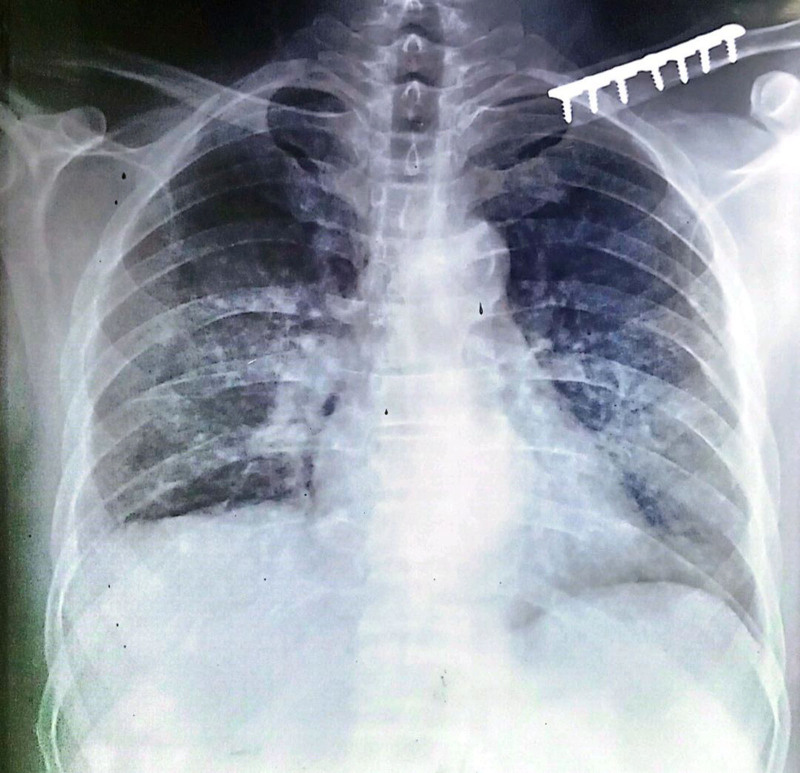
Chest x-ray (PA view) shows bilateral heterogeneous infiltrates and right sided pleural effusion. x-ray = radiograph.
